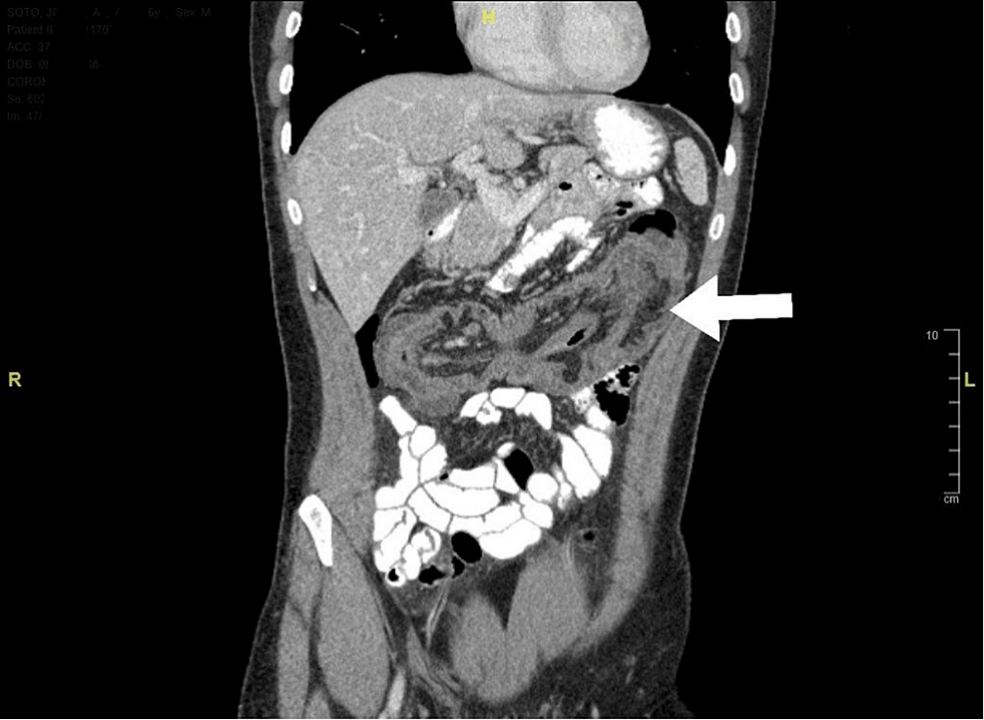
CT scan of the abdomen and pelvis without contrast (coronal view) showing long segment intussusception marked by the arrow
